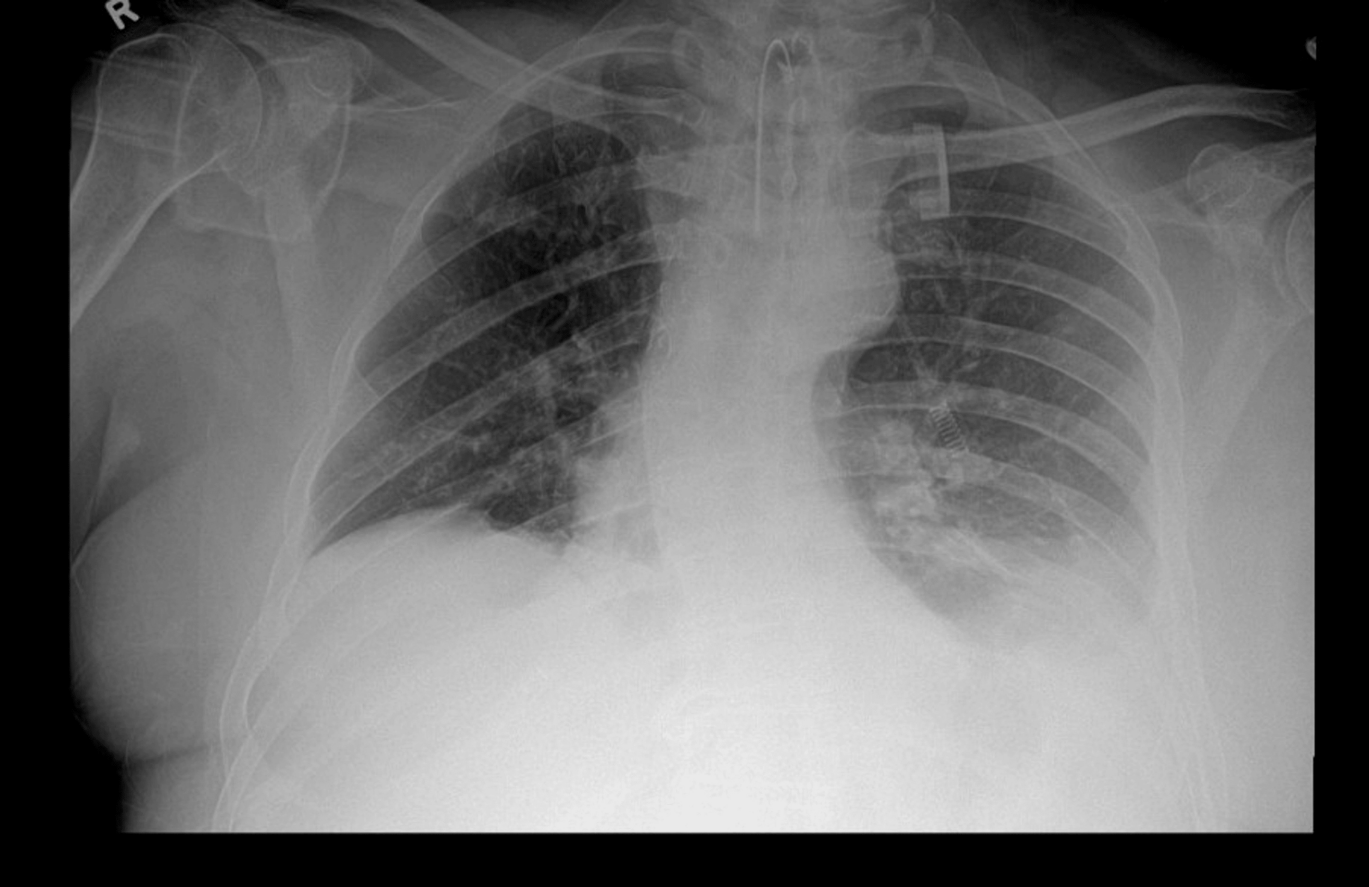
Chest radiograph demonstrating bilateral lower lobe atelectasis with elevated right hemidiaphragm and mild scoliosis, consistent with respiratory compromise in a patient with Charcot-Marie-Tooth disease.
